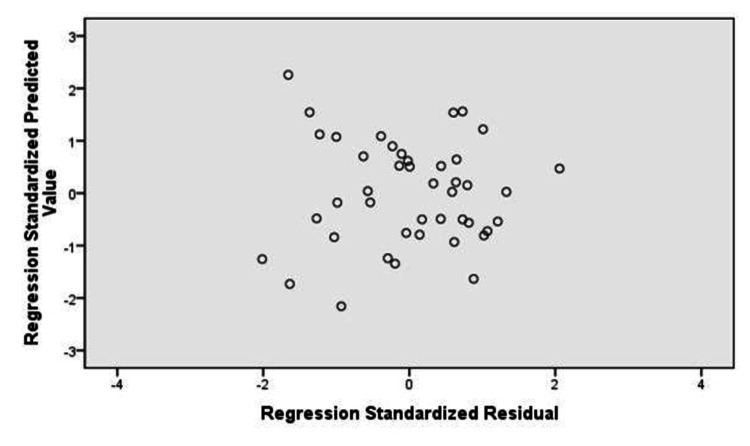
Scatter plot Dependent variable %BF US %BF, body fat percentage; US, ultrasound
